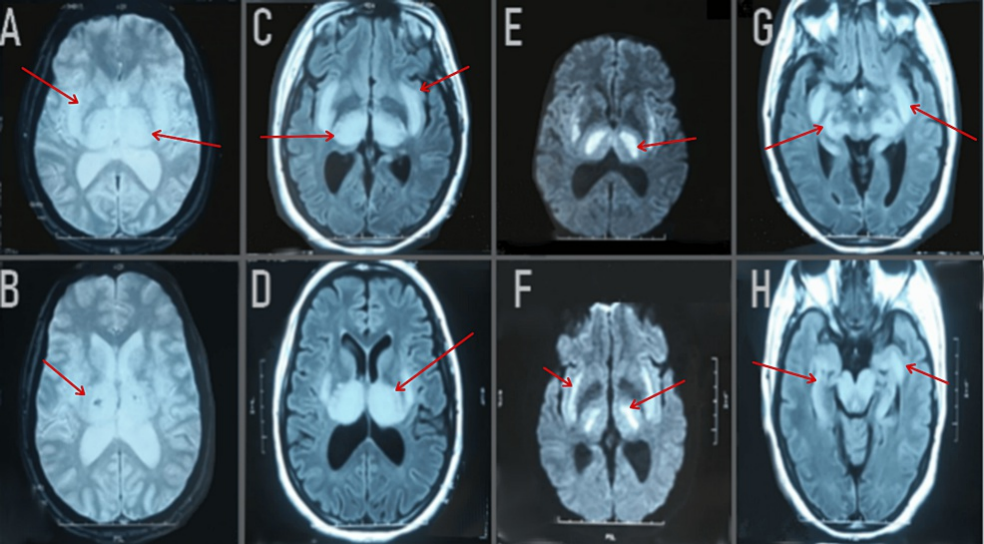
Axial FLAIR images (C, D, G, H) showed bilateral hypersignal abnormalities (red arrow) in the thalamus, posterior limb of the internal capsule, external capsule, putamen, hypothalamus, pons, and bulb. Axial images with gadolinium demonstrated no abnormal contrast enhancement (not shown). T2*-weighted images (A, B) showed bilateral thalamic hemorrhagic lesions. Diffusion-weighted imaging (E, F) showed a hypersignal B1000 with an apparent diffusion coefficient decrease. FLAIR: Fluid-Attenuated Inversion Recovery; T2: T2-weighted imaging; DWI: Diffusion Weighted Imaging; ADC: Apparent Diffusion Coefficient.
